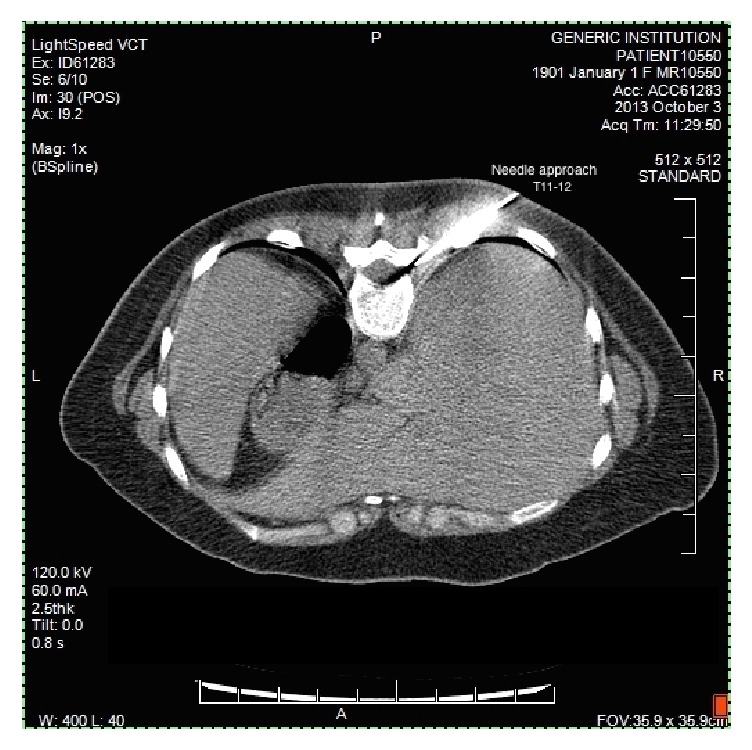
CT-guided image displaying the Tuohy needle trajectory to achieve right-sided transforaminal epidural placement for the injection of hydrogel sealant.
